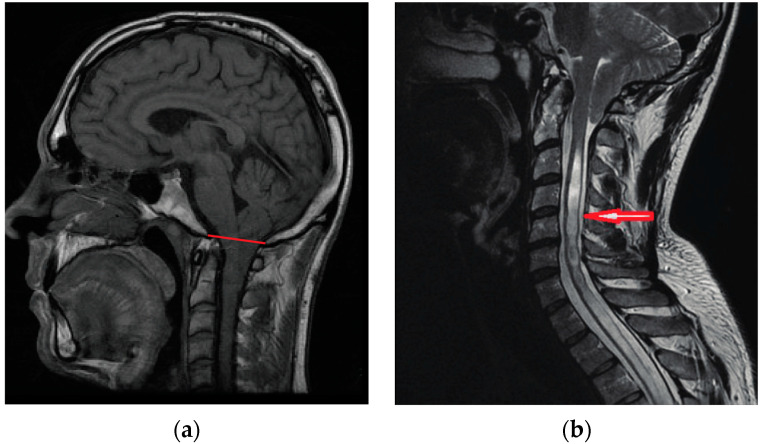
Chiari 1 Malformation (CM1). Sagittal MRI (a) Male, 24 years; CM1 is defined as a displacement of the cerebellar tonsils greater than five millimeters below the basion–opisthion line (red line); (b) Female, 38 years; CM1 with long segment syringomyelia (red arrow).
